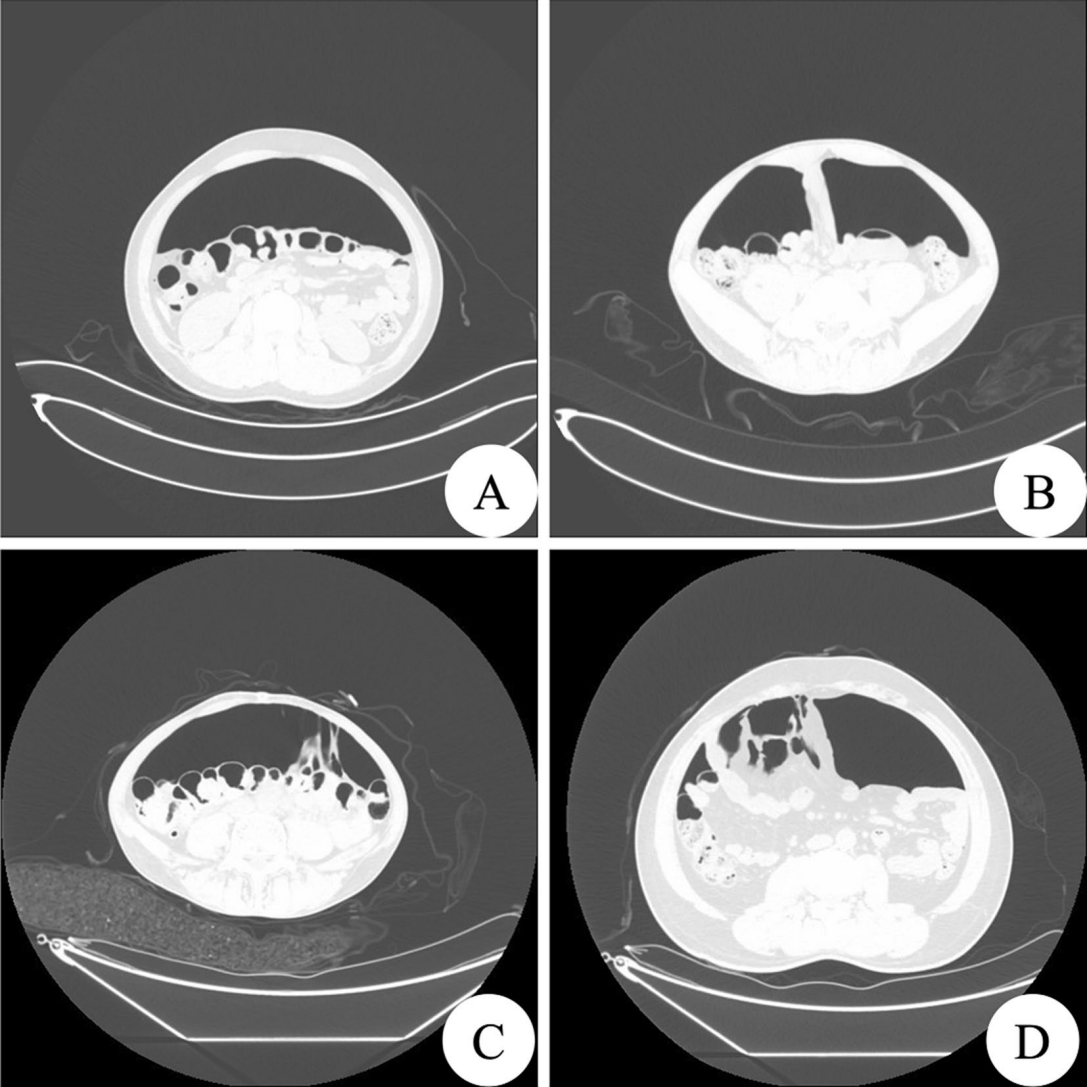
Image of the graded diagnosis of intestinal adhesions on CT with pneumoperitoneum. (A) Shows the CT image of the abdomen after pneumoperitoneum as level 0. (B) Shows the CT grade diagnosis of pneumoperitoneum as level 1. There is a single point adhesion between the local small bowel tube and the anterior abdominal wall. (C) Shows that the CT classification of pneumoperitoneum is level 2, showing that part of the intestinal tube and omentum tissue has multiple adhesions to the anterior abdominal wall. (D) Shows that the CT classification of pneumoperitoneum is level 3, and some diffuse adhesions of the anterior abdominal wall of the intestine and omentum tissue can be seen.
